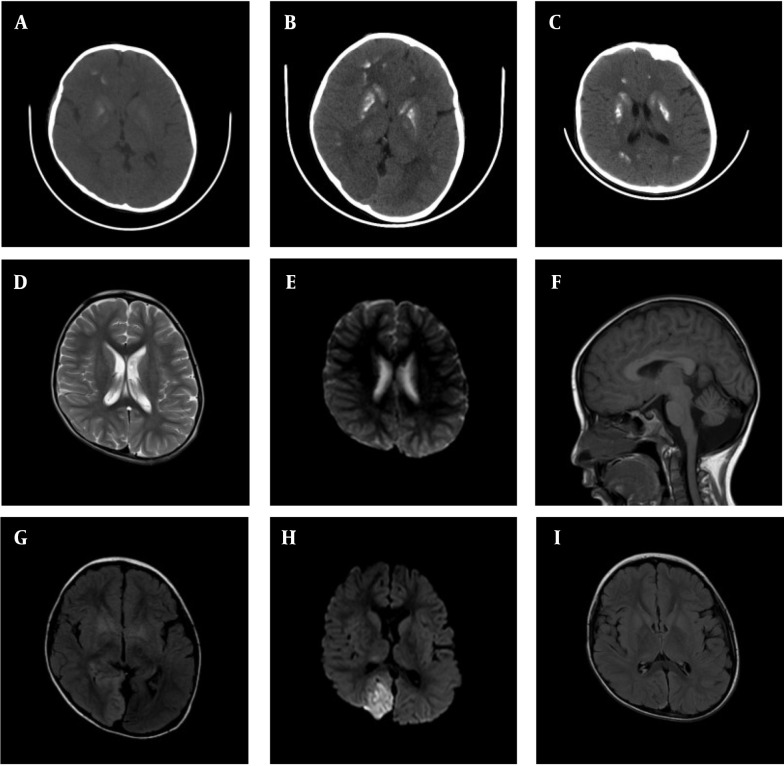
A – C, Brain CT scans obtained one year ago, during the first hospitalization, and at the second hospitalization after the current onset, respectively. Extensive basal ganglia calcification is observed, predominantly affecting the striatum and globus pallidus. Over the past year, the degree of calcification has increased markedly, though recent changes are relatively unremarkable; D and E, head MRI results from the local hospital in 2023, showing no significant abnormal high signals in T2 and DWI sequences; F - H, head MRI results during the first hospitalization in the current onset, showing patchy high-signal areas on T2 and FLAIR sequences, along with high signals on DWI in the right parieto-occipital lobe. Slight cerebellar atrophy is also noted; I, Head MRI results from the second hospitalization during the current onset, showing slight abnormal signals in the right parieto-occipital lobe, with a significant reduction in the extent compared to the previous hospitalization.
